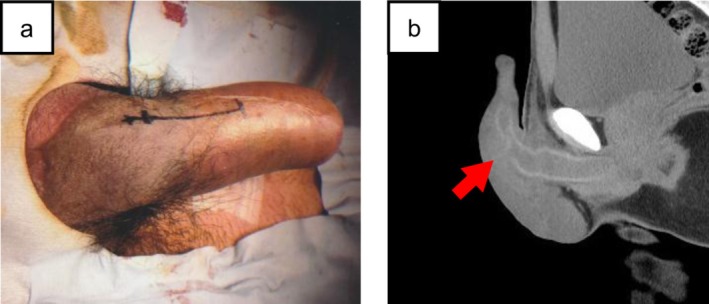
(a) Preoperative image of the penis with subcutaneous hematoma. (b) Computed tomography (CT) image demonstrating a laceration of the cavernosal tunica albuginea.
